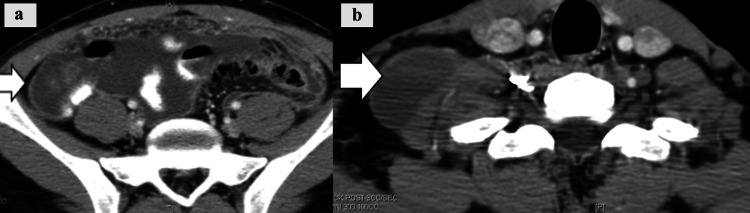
Tuberculous peritonitis. a: CT image shows thickening of omentum (white arrow) with ascites (wet type of TB); b: CT image of the neck shows right supraclavicular lymphadenopathy (white arrow) secondary to TB in the same patient.
